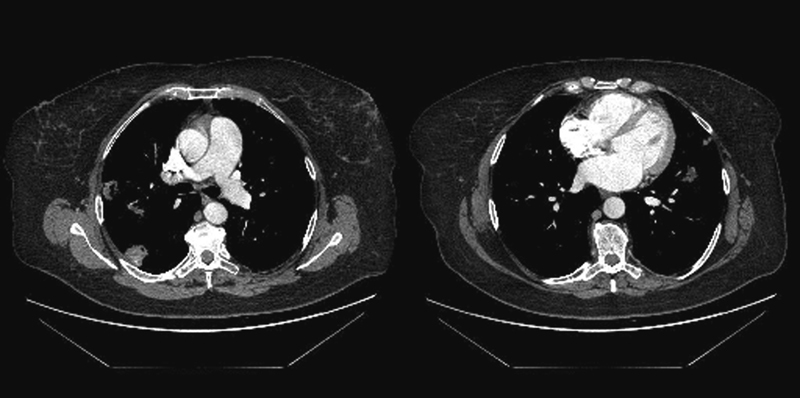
Thoracic computed tomography with contrast media. (Left) The calcified lesions with lobulated contours in the right lobe of lungs. (Right) The lesion in the left lobe of lungs.
